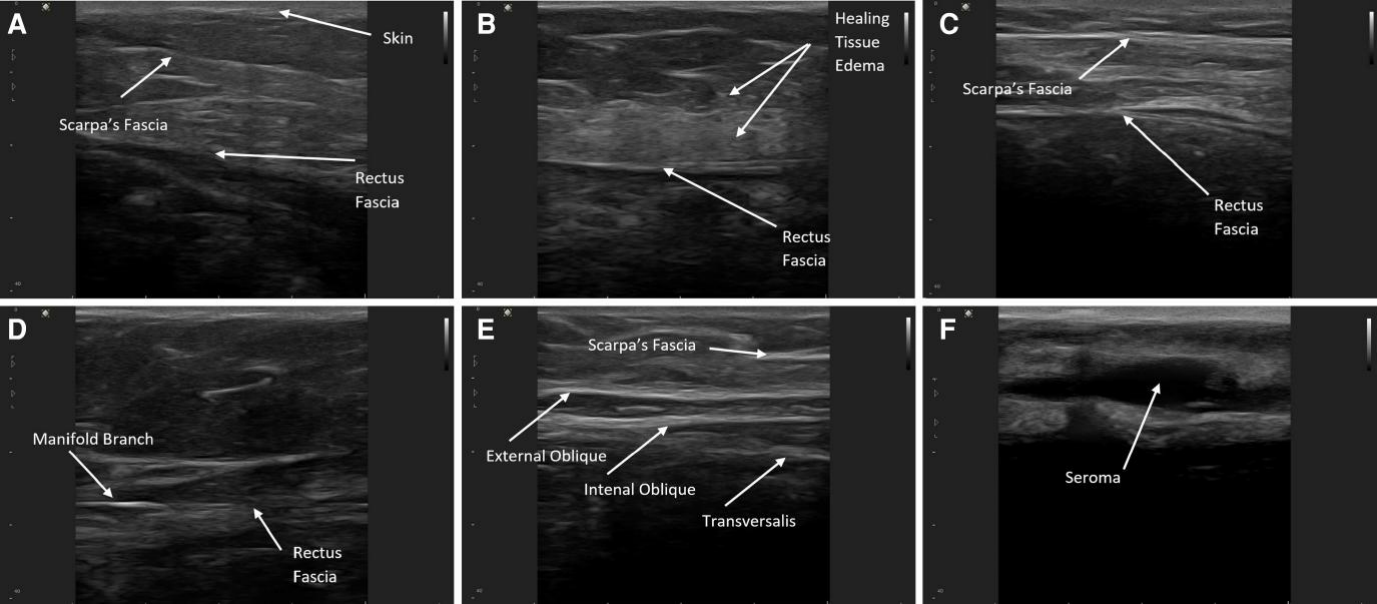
(A-E) Sample high-resolution ultrasound (HRUS) images (Plastic Surgery Imaging—12 MHz transducer) from the first 71 consecutive Interi system (Internal Closure System, IC Surgical, Grand Rapids, MI) patients recorded at the time of device removal showing no fluid collection with documented anatomy. Skin, Scarpa's fascia, and abdominal fascia are marked. (F) One of the 3 postoperative patients that developed a seroma. She failed to activate the new therapy unit on postoperative Day 3.
